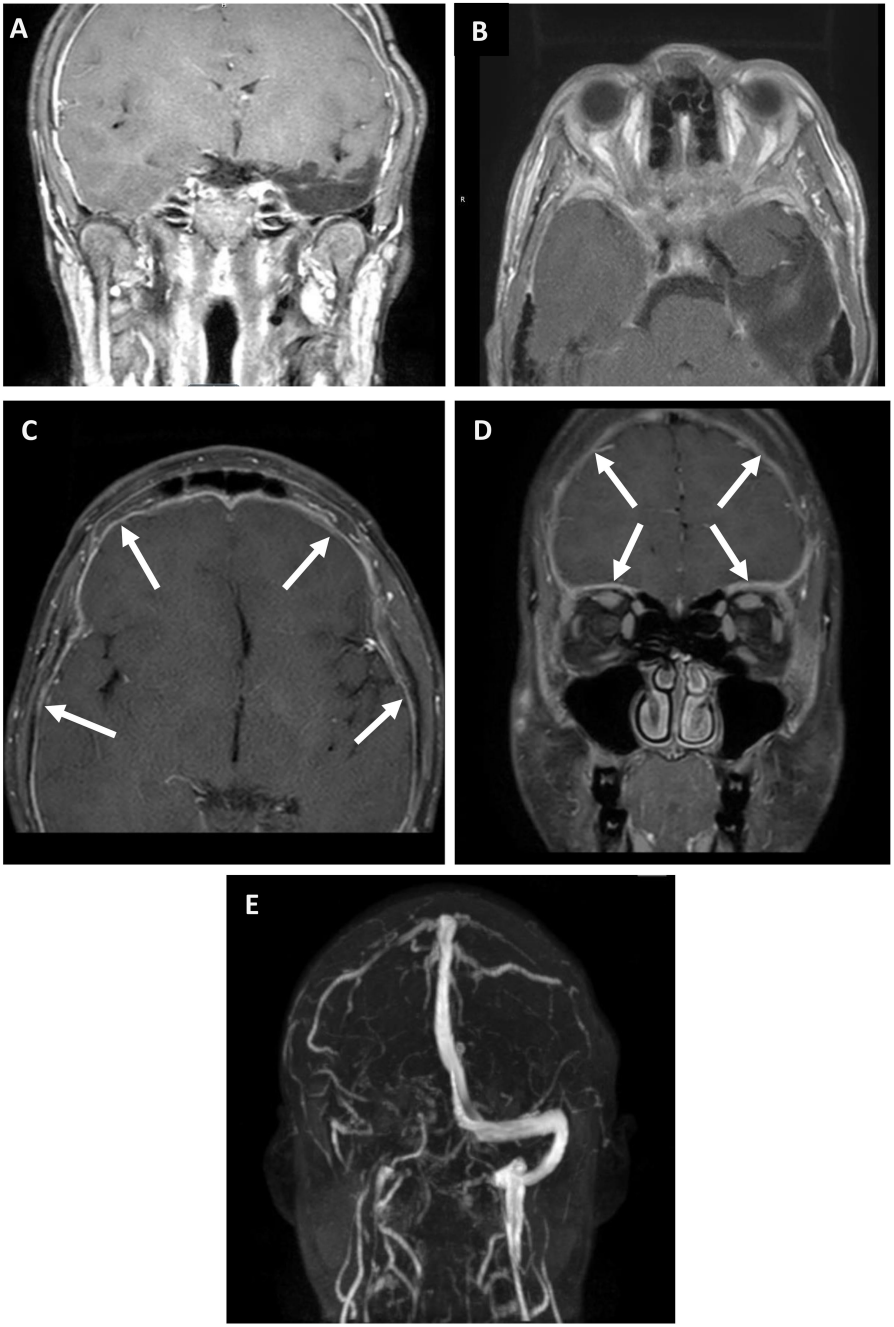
MRI/MRV brain and orbit scans with gadolinium at presentation. (A–D), Axial and coronal T1-weighted postcontrast fat-saturated images, 13 years after a left temporal lobe tumor resection, which demonstrate postoperative changes with diffuse, smooth dural enhancement over the frontal and temporal lobes (arrowheads). (E), Coronal 2D TOF MRV scan showing a congenitally hypoplastic right transverse sinus, and, to a lesser extent, the right sigmoid sinus and the proximal right jugular vein. There was no evidence of a dural sinus thrombosis or findings that were suggestive of an elevated intracranial pressure on neuroimaging.
